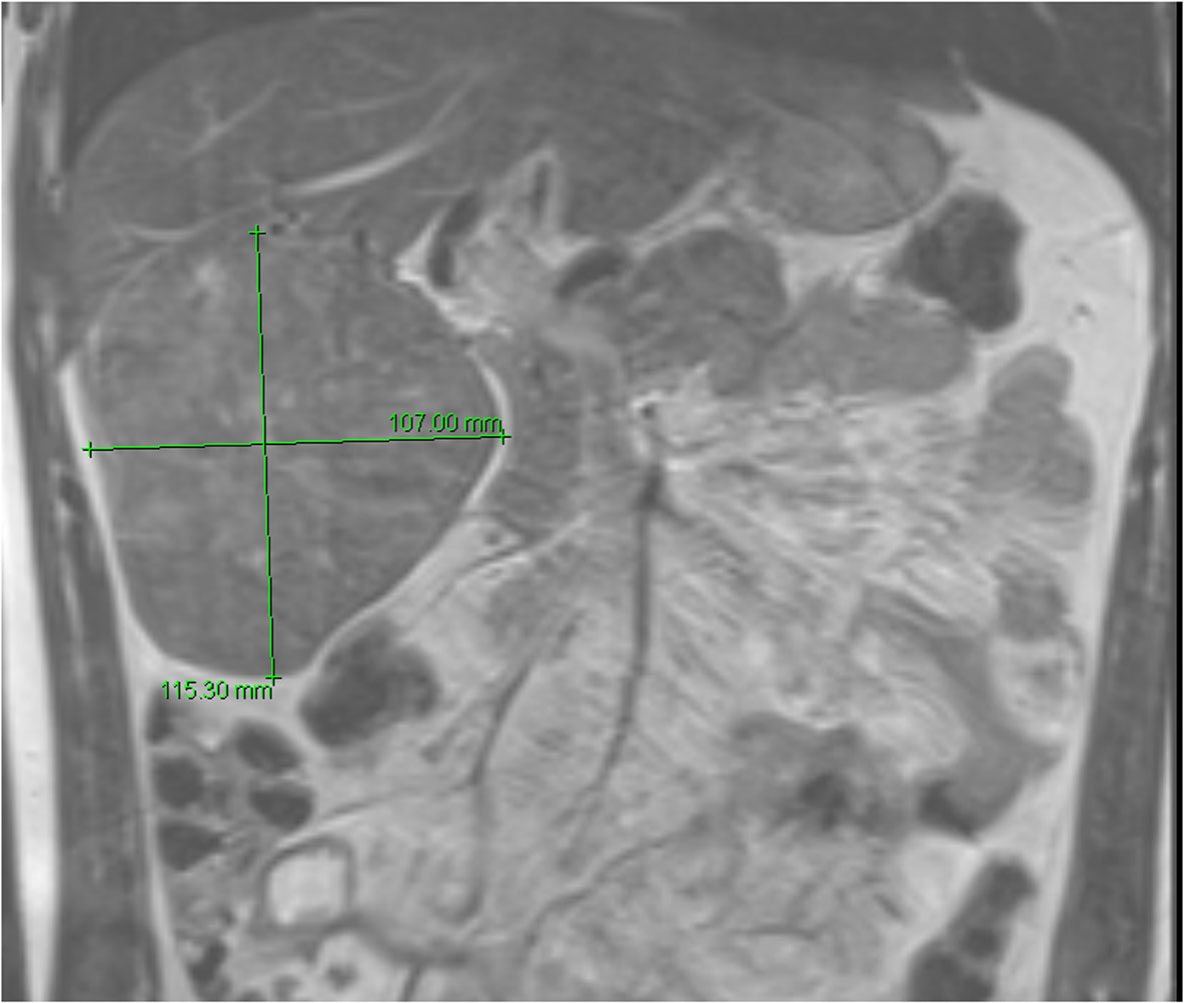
T1 weighted MRI showing a 10 × 11 cm mass in the right liver lobe
